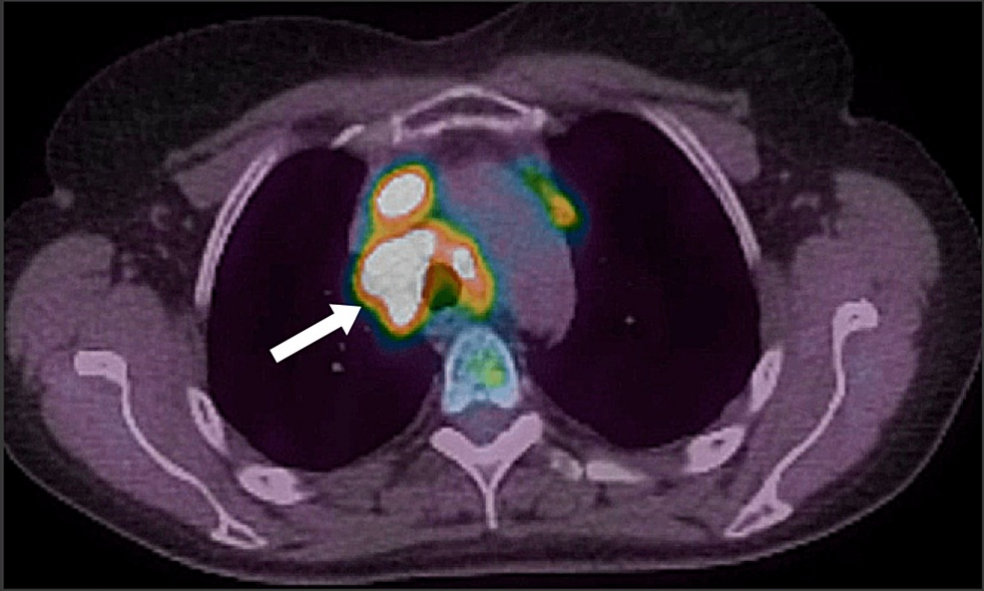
PET scan of mediastinal and hilar lymph nodes with high FDG-avidity (subcarinal lymph node - SUV of 15.7)
